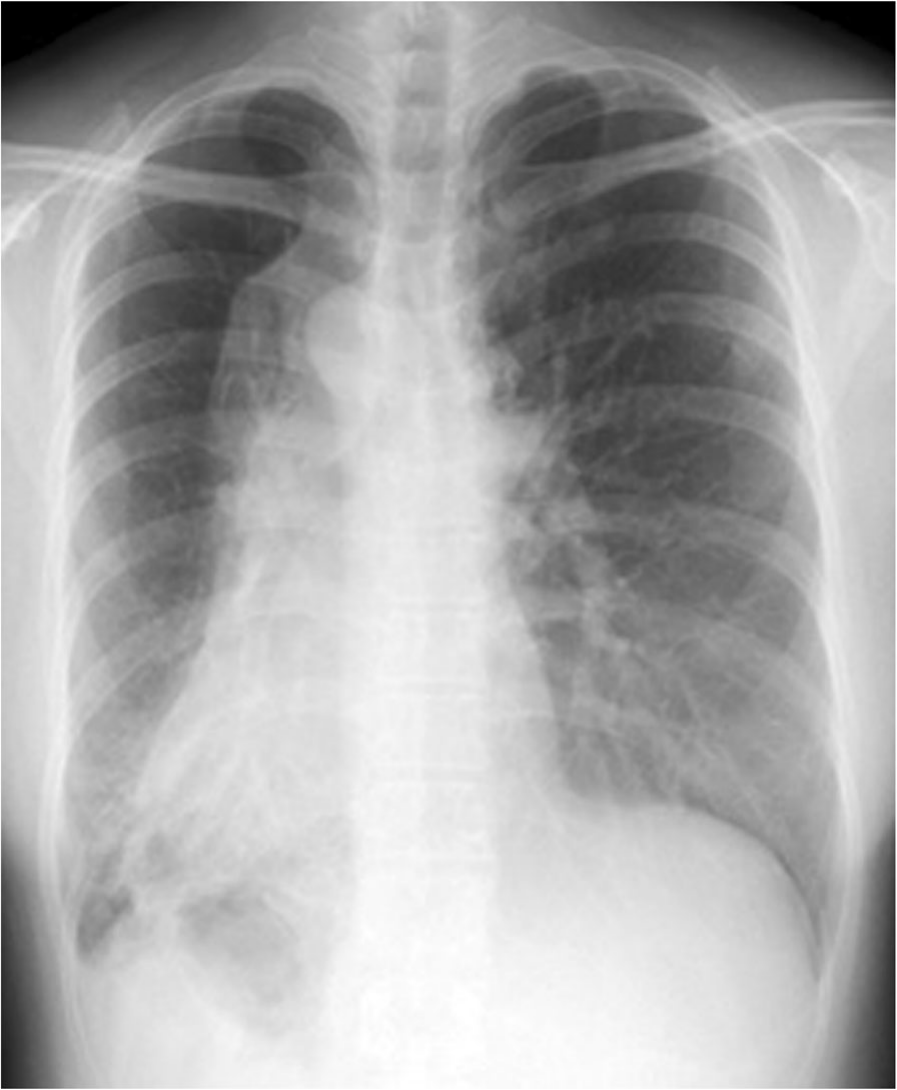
Chest X-ray examination performed on admission. Chest X-ray image showing an infiltrative shadow in the right upper lung field suggesting atelectasis of the right upper lobe and tram lines with nodular shadows in both lung fields
